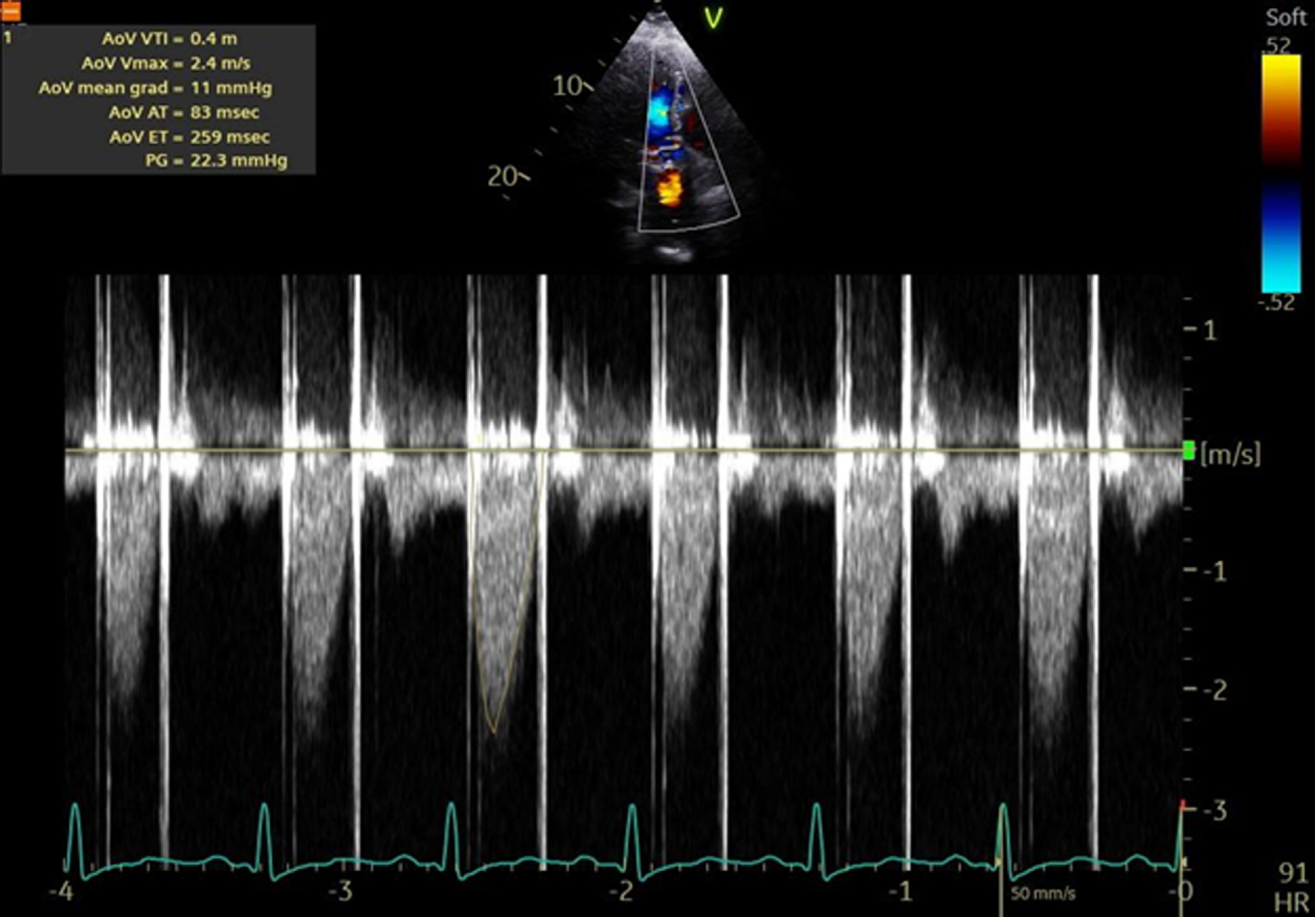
Cardiac Computed Tomography with Intravenous Contrast Cardiac computed tomography was obtained for better characterization of the valve and to rule out any thrombosis. The image shows areas of hypoattenuation on the ventricular side of the mechanical aortic valve. The mean Hounsfield units of both areas as marked by the circles (41.1 and 63.3) are much lower than the circle marking the aortic area (178) concerning for a clot/thrombus of the mechanical aortic valve.
